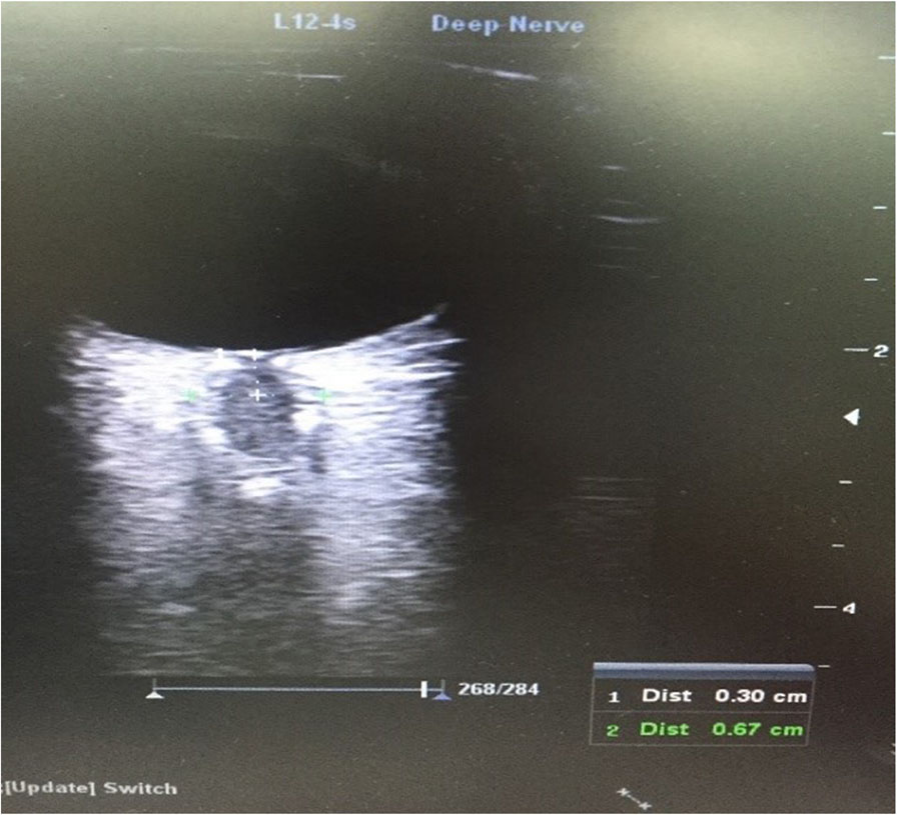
Ultrasonographic ONSD measurement
